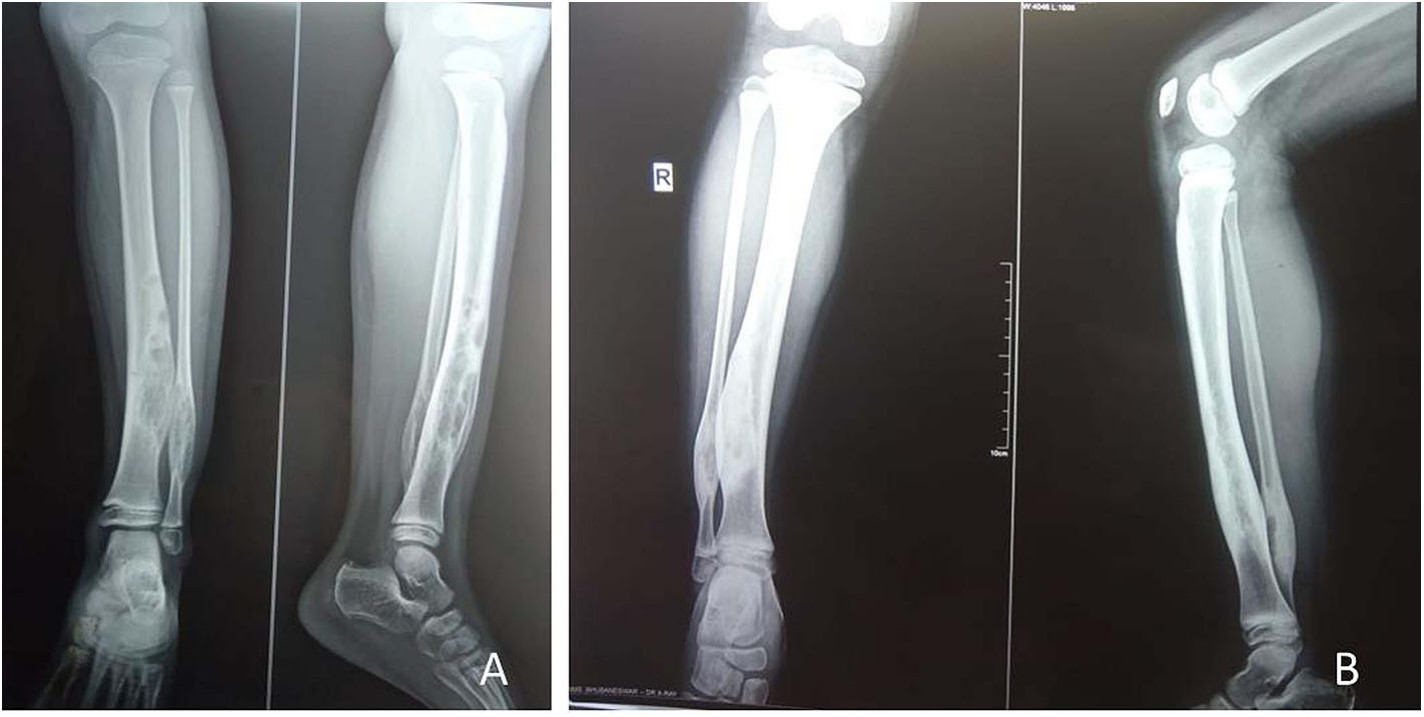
A 5-year-old female child with polyostotic fibrous dysplasia (PFD) of tibia, fibula (A); follow up radiograph at 6 months revealed complete healing of the lesion (B).
